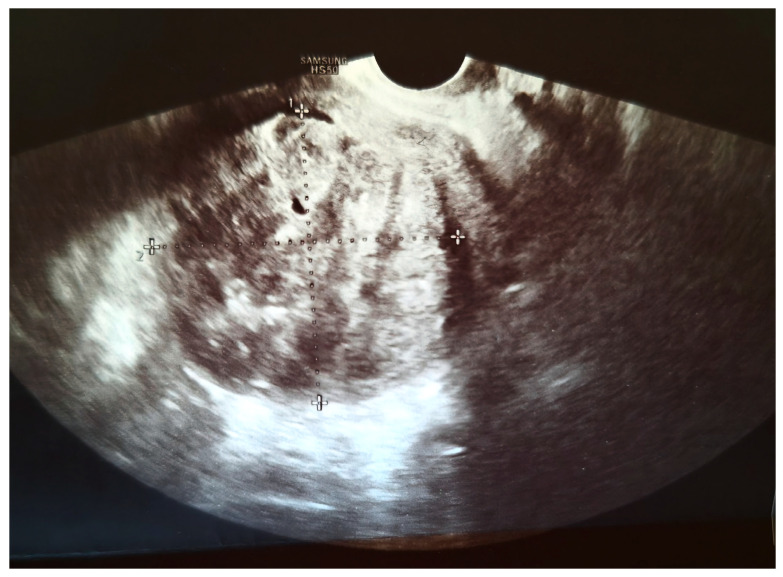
Transvaginal ultrasound image (transverse plane) showing a uterine mass measuring 64 × 62 mm.
